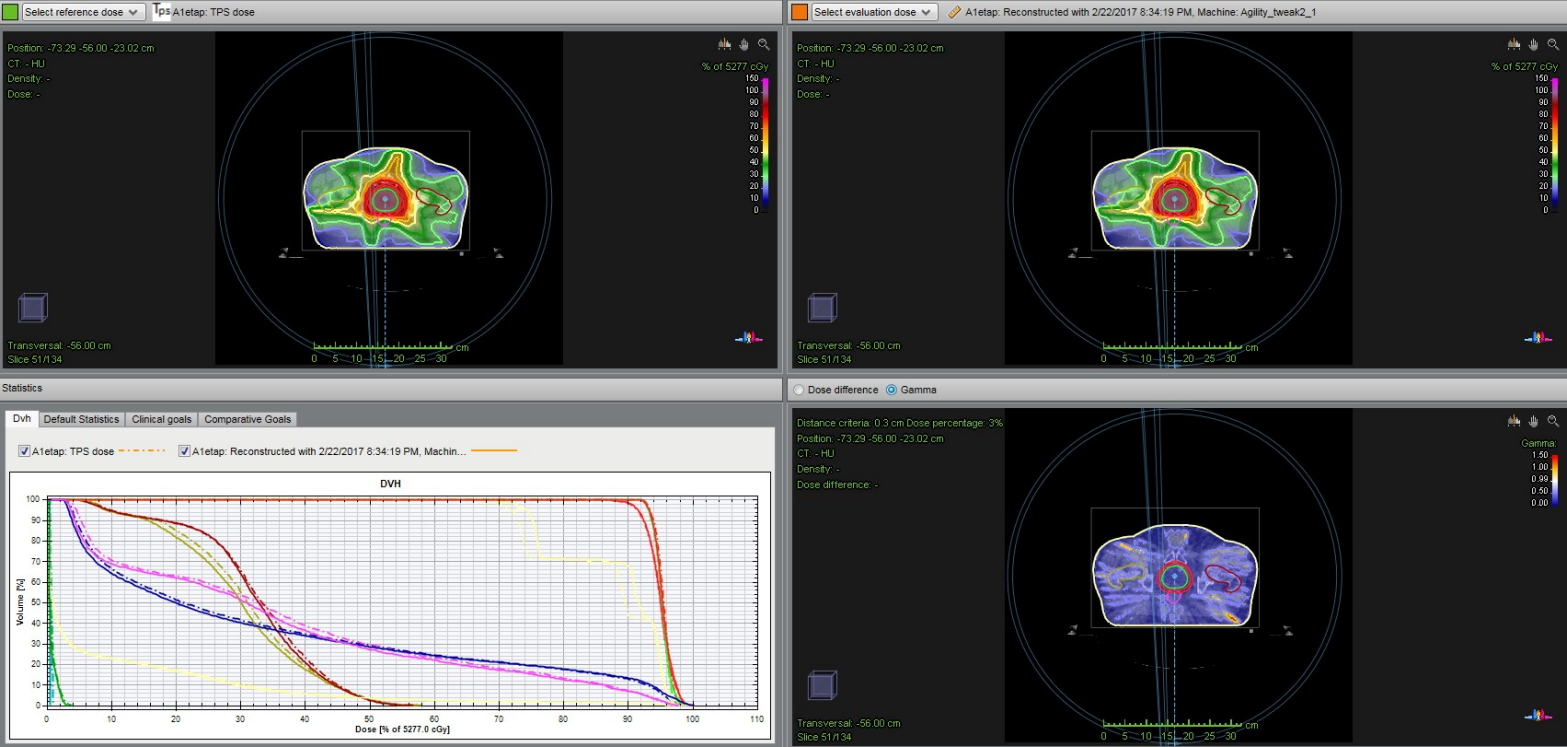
3D gamma index (GI) analysis in the Compass system on a patient’s CT scan with evaluation of DVH_Compass_ versus DVH_TPS_ in a patient with prostate cancer.
